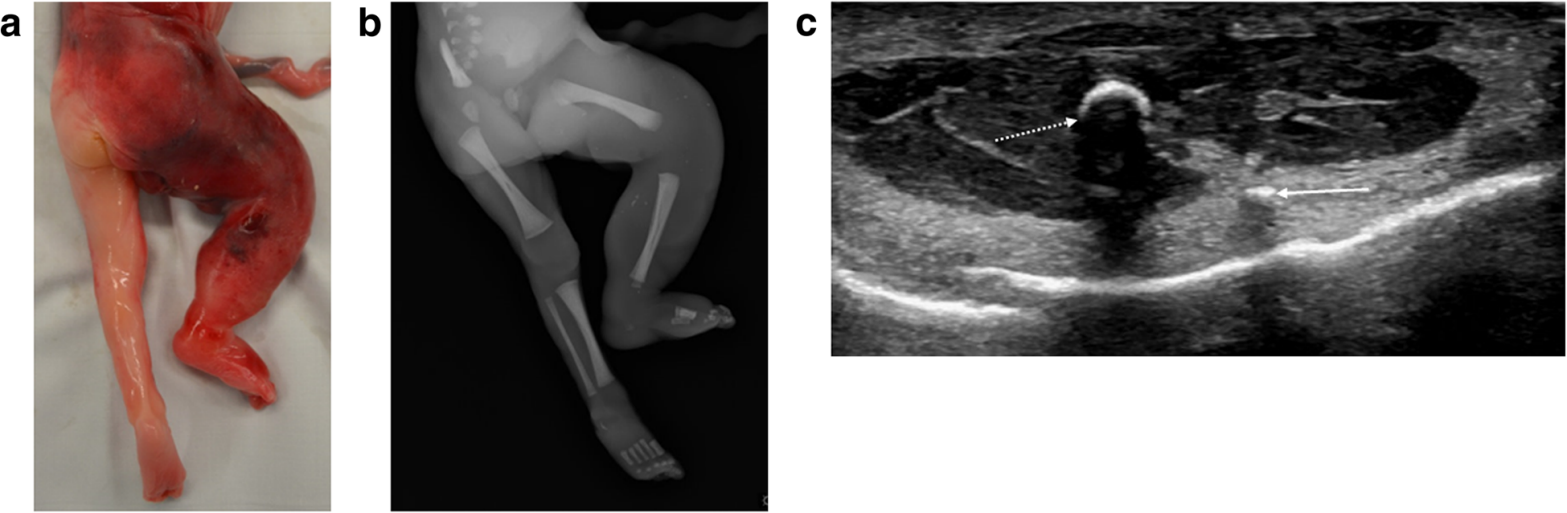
a–c Klippel-Trenaunay syndrome in a foetus at 22 weeks gestation, following termination of pregnancy. The external photograph of the lower limbs at autopsy (a), seen from behind, reveals an enlarged right leg with discoloured red and purple overlying skin. The post-mortem lateral radiograph of this right limb (b) reveals numerous small hyperdense foci within the soft tissues which are tiny phleboliths. At post-mortem ultrasound of the right thigh (c), obtained in axial plane 1 day after delivery, the small phleboliths were well seen with posterior acoustic shadowing. One of these is demonstrated with a solid arrow, the dotted arrow represents the right femur. At autopsy, extensive ectatic thin-walled vessels containing thrombus were seen in the right leg and in keeping with a vascular malformation. Due to lack of circulation and small diameter of vessels, this vascular anomaly could not be well depicted on ultrasound
